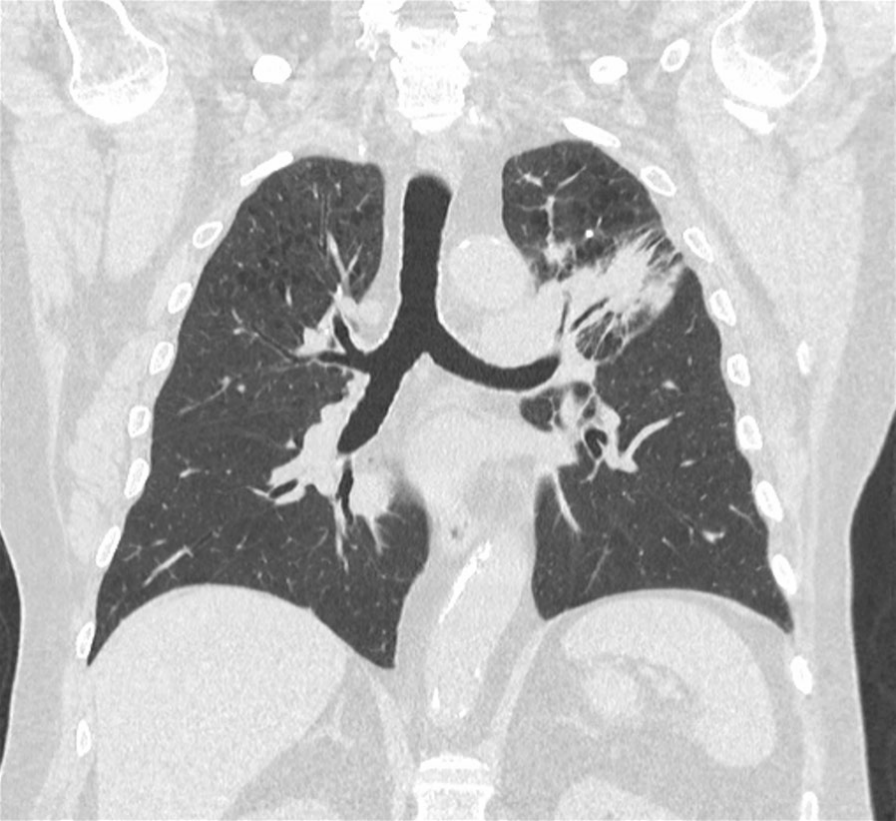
Case 3—CT chest before immunotherapy commenced
